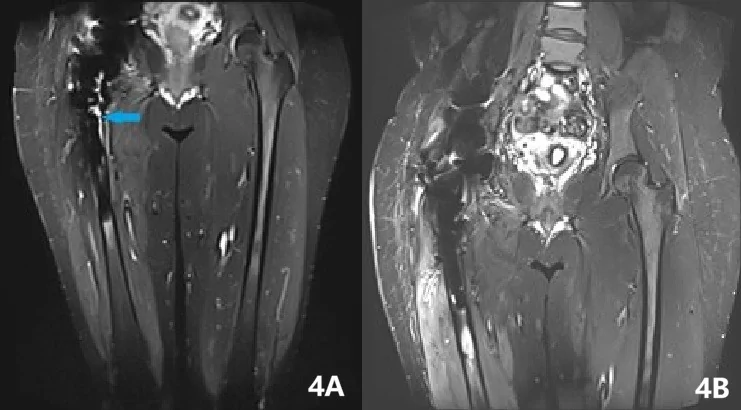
a) Preoperative metal artifact reduction sequence (MARS) sequence of the right hip debridement, with metal-bone interface infection manifestations indicated by the blue arrow. b) Review MRI MARS sequence three months after debridement.
